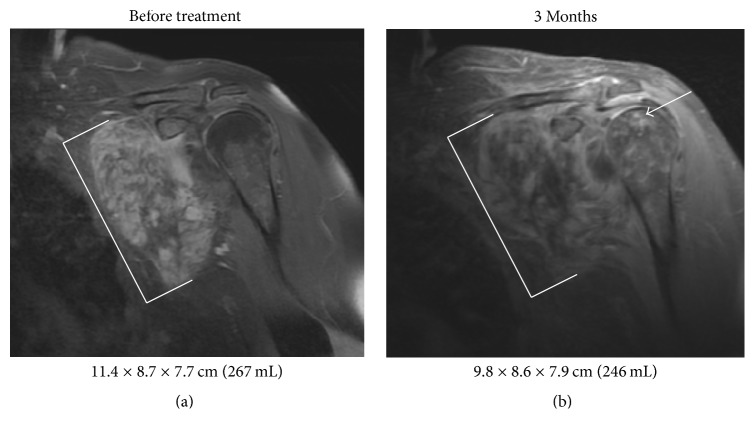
Imaging of Axillary Desmoid Treatment Response. Coronal T1 postcontrast MRI of a left axillary desmoid tumor before (a) and 3 months after (b) completing radiation therapy to 50.4 Gy in 28 fractions with concurrent imatinib demonstrating decreased enhancement with minimal change in tumor size. The tumor is delineated with white brackets. Imaging changes noted in the humeral head compatible with avascular necrosis are indicated with the white arrow.
